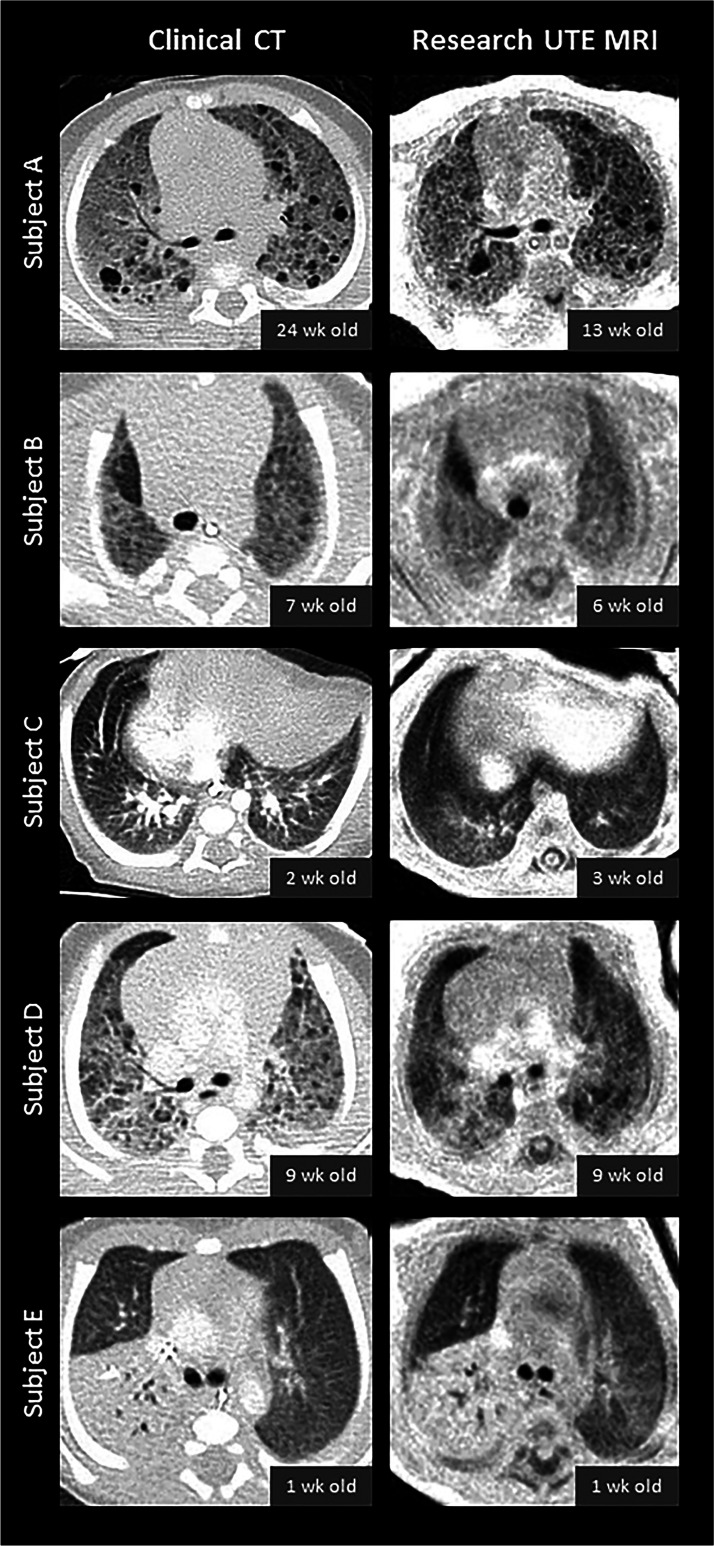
Comparison of five neonates’ matched axial CT and axial ultrafast echo-time (UTE) MRI slices obtained at a similar time visually demonstrates the similarity of tissue density and lung findings. Subject A is a neonate with severe bronchopulmonary dysplasia (BPD). The quantitative density of UTE is highly correlated with CT density in the lung. Note the different appearance in the spinal canal on the MRI caused by the different T1 and T2^*^ properties in the spinal canal compared to the lung tissue. Reproduced with permission [64]
